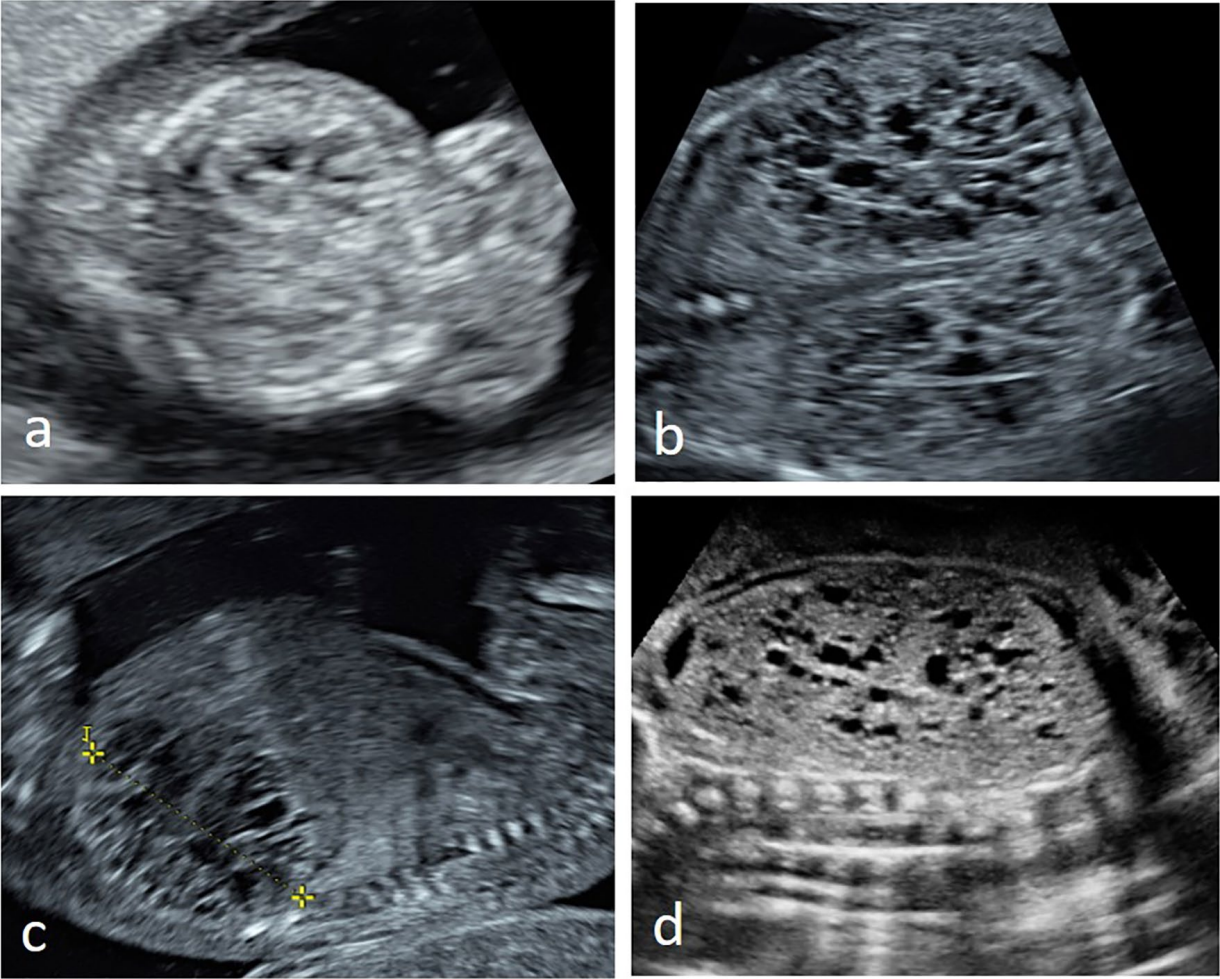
Prenatal ultrasound of typical polycystic and dysplastic kidneys in two fetuses with Meckel–Gruber syndrome at a 12 + 0 weeks and b + c at 14 + 5 weeks; note the premature (“too advanced”) CMD in first trimester and the missing CMD later in pregnancy; kidney cysts are small, numerous and intersperse in the entire renal parenchyma with pronounced occurrence in the medulla
